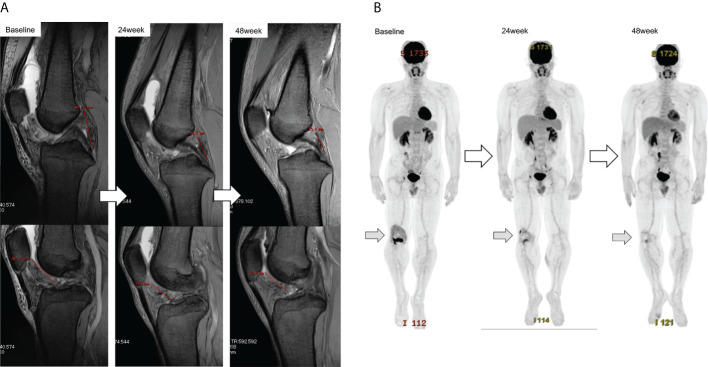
Response of the target tumor size on MRI in patient ZLT-01-08 (A). The longest dimensions judged by the local investigator were 41.5 mm and 40.2 mm at baseline, 30.5 mm and 31.6 mm at 24 weeks, and 25.6 mm and 33.9 mm at 48 weeks. The reduction rates were 15.4% at 24 weeks and 10% at 48 weeks, as judged by the local investigator, and 31.4% at 24 weeks and 30.5% at 48 weeks, as judged by the central committee radiologist. Metabolic response of the target tumor size on FDG-PET in patient ZLT-01-08 (B). Reduction rates were 59.2% at 24 weeks and 70.4% at 48 weeks. MRI, magnetic resonance imaging; FDG-PET, 18F-fluorodeoxyglucose-positron emission tomography.
